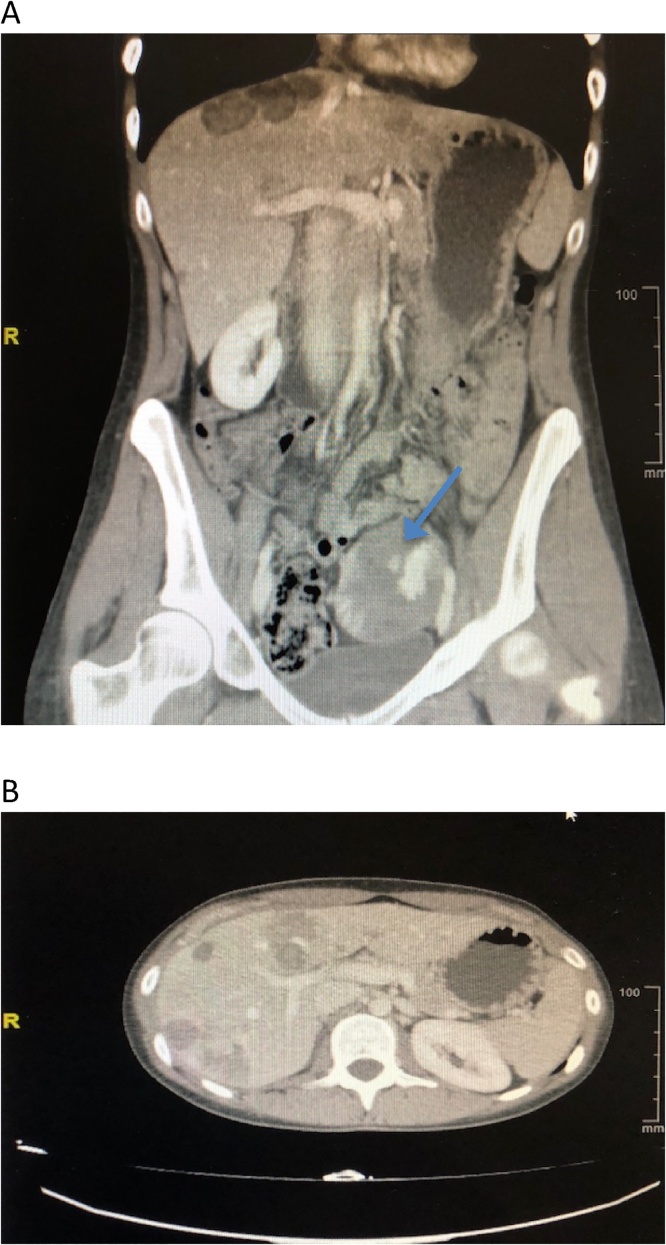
Abdominal CT scan during the portal phase. (A) Pelvic mass (blue arrow). (B) Multiple hepatic lesions.
